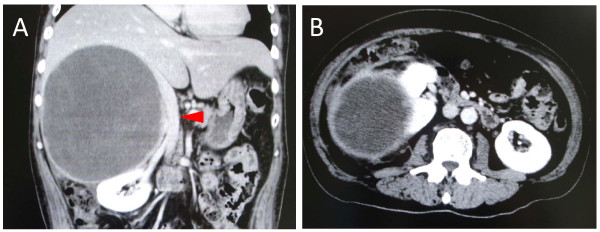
An enhanced computed tomography of the abdomen revealed a giant homogeneous low density mass lesion in the right adrenal region. (A) A giant mass lesion was indented over the inferior aspect of the right lobe of the liver and a displaced inferior vena cava (arrowhead) with no abdominal lymphadenopathy. (B) The right kidney was also ventrally displaced.
